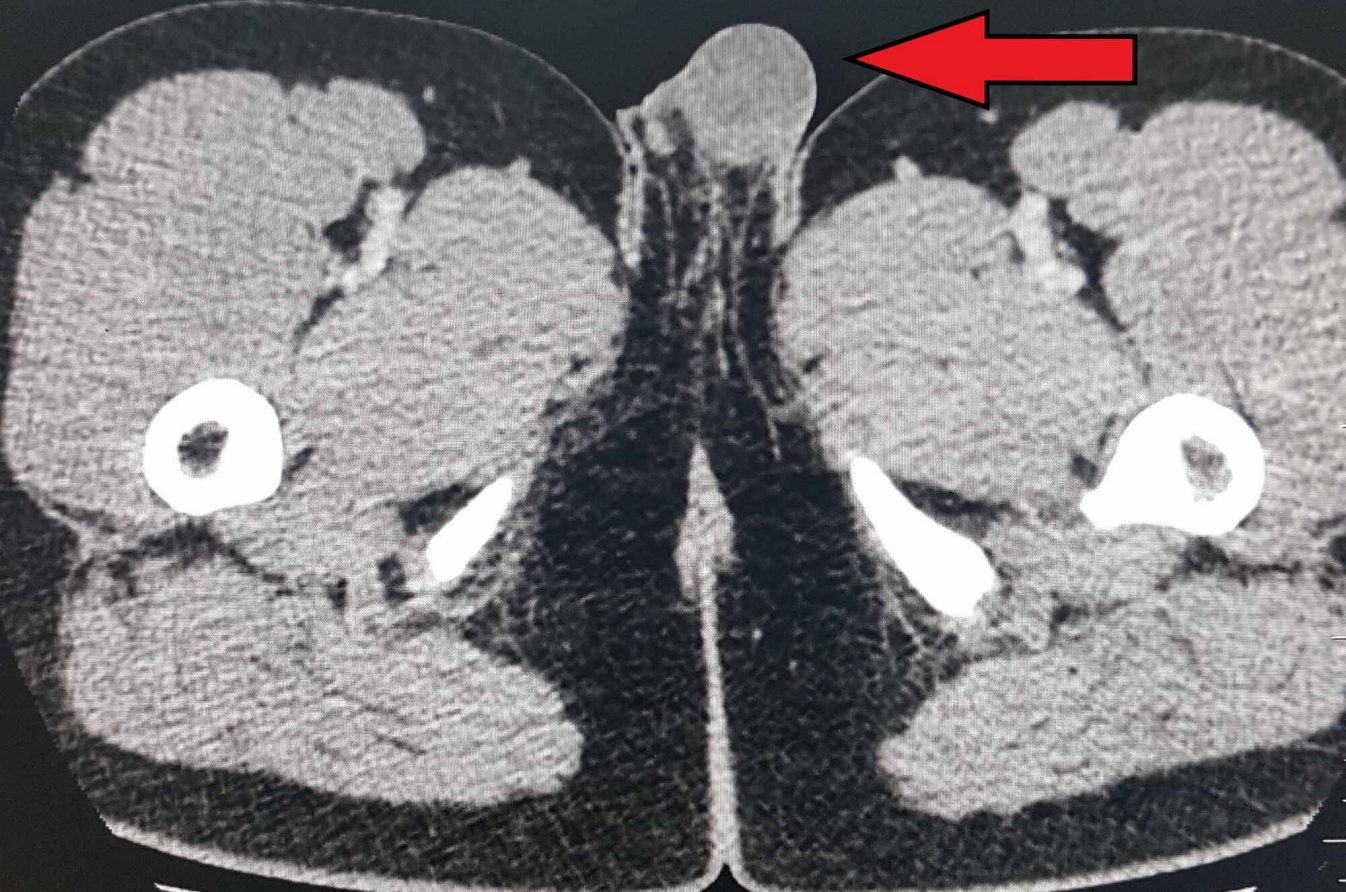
Left testis is visualized in the scrotum with agenesis of the right testis on CT.
